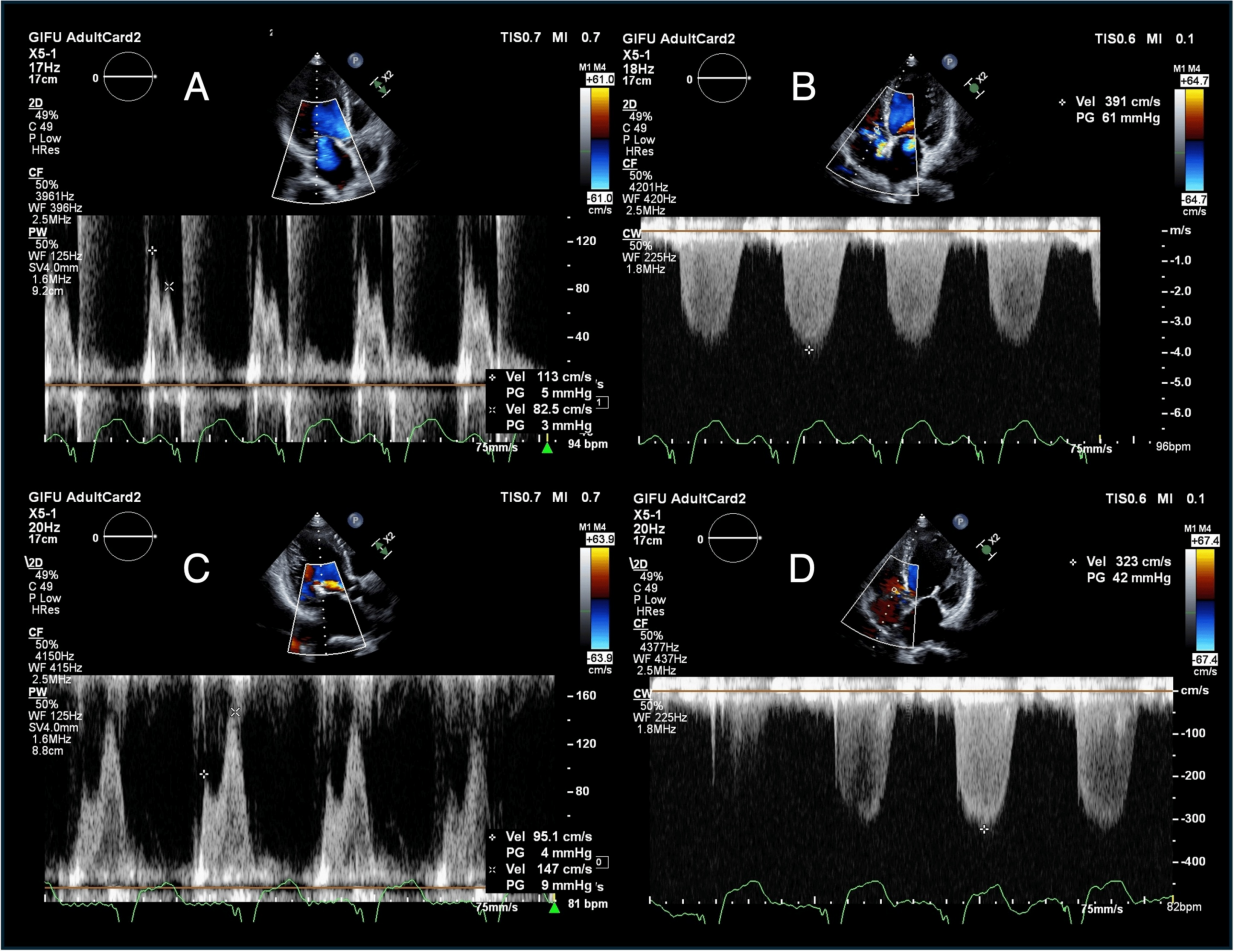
Exercise stress echocardiography at three minutes post-exercise, pre- and post-treatment Pre-treatment: A: transmitral flow (E/A 1.4); B: TRPG 61 mmHg, vitals: BP 126/70 mmHg, HR 96 bpm; Post-treatment: C: transmitral flow (E/A 0.65); D: TRPG 42 mmHg, vitals: BP 112/59 mmHg, HR 80 bpm At peak exercise post treatment, the patient exhibited E/A 1.0, TRPG 57 mmHg (PASP 67 mmHg), and moderate MR, indicating exercise-induced pulmonary hypertension. However, as shown, hemodynamic recovery was faster post-treatment. TRPG: tricuspid regurgitation pressure gradient; PASP: pulmonary artery systolic pressure; MR: mitral regurgitation; BP: blood pressure; HR: heart rate; bpm: beats per minute
